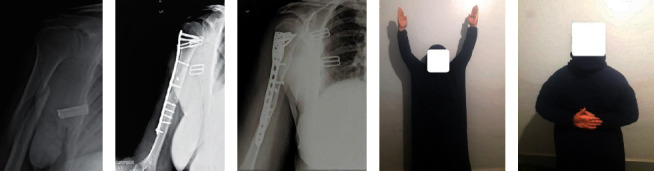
A 41-year-old female patient had humeral diaphyseal fracture due to a car accident. Preoperative X-ray shows AO/OTA type 12-B_1_ (a). She was treated by MIPO technique with complete fracture union as shown in 5 months postoperative X-ray (b, c). The patient had full shoulder function and complete range of motion (d, e).
